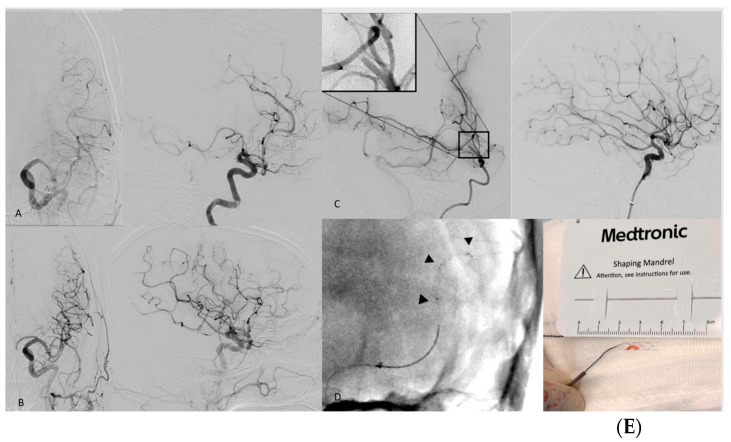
(A,B) Angiographic images of a 64-year-old stroke patient with an occlusion of the inferior trunk of the M2 segment on the left side; image B shows the result after recanalization eTICI 3. (C) M2 occlusion and deficit on lateral view of angiography with magnification of the occlusion. (D). 3D reconstruction using flat-detector CTA. (C) mTICI 3 result after successful EVT. (D) Highly visible Solitaire X 3 mm SR. (E) Ex-vivo photography of the Solitaire-X and a captured thrombus (Image curtesy of Dr. Lüttich).
